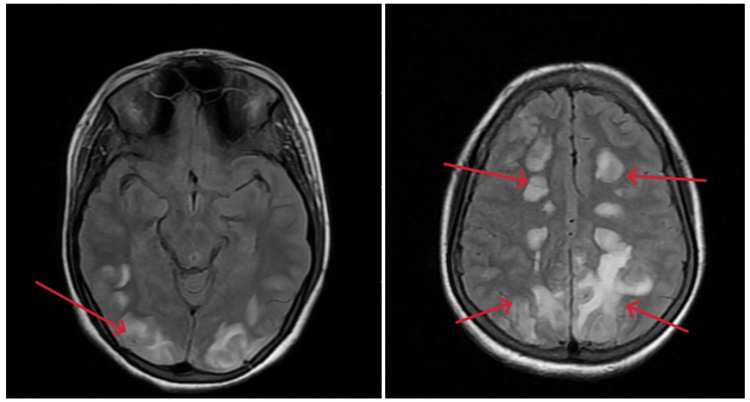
MRI of the brain (axial section) showing bilateral symmetrical altered signal intensity (red arrows) in the subcortical white matter of the occipital and parietal lobes, extending to the frontal lobes, on T2/FLAIR-weighted imaging. FLAIR, fluid-attenuated inversion recovery
